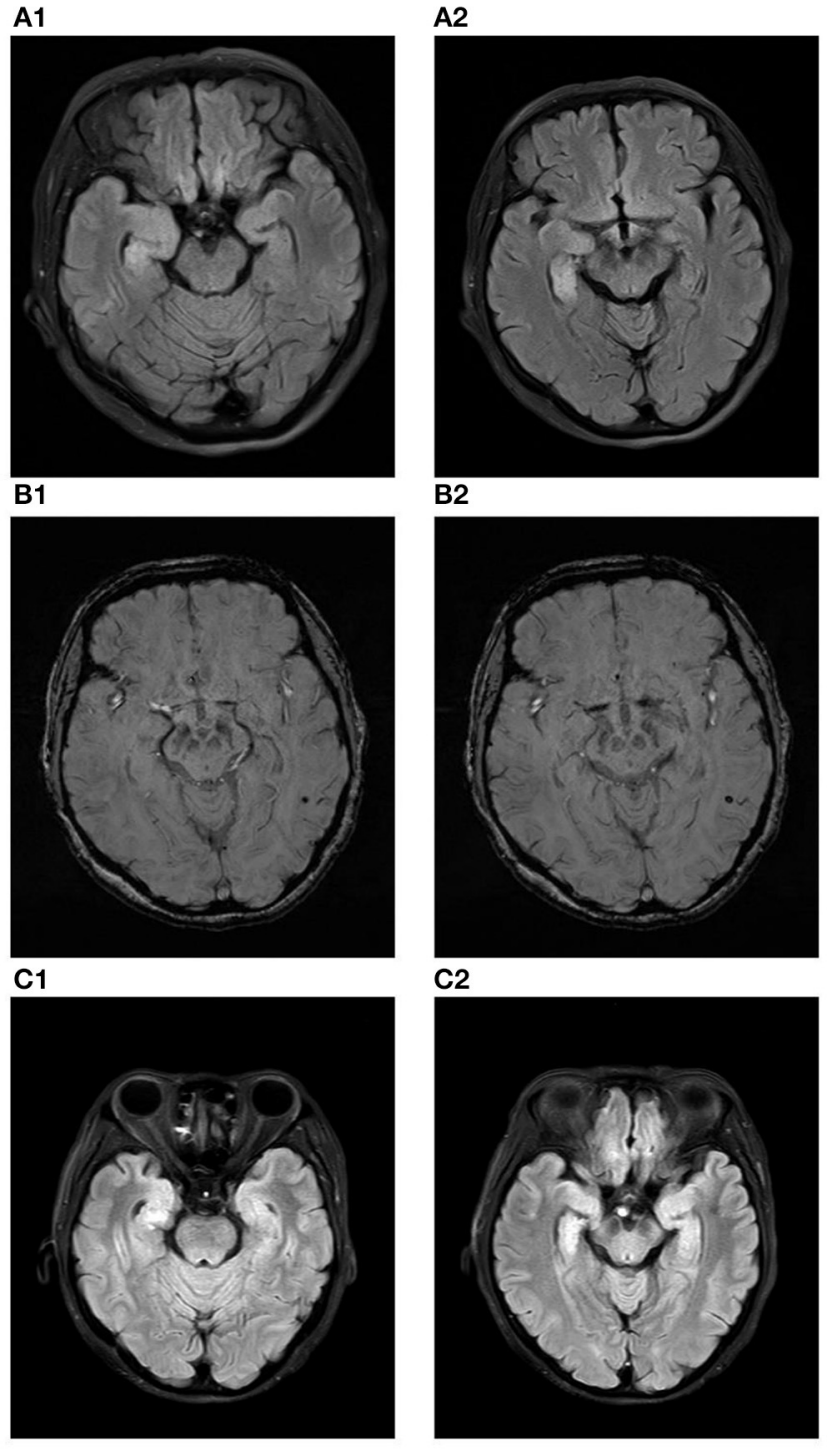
(A1,A2) show the increased volume of the right hippocampus and hyperintensities in the fluid attenuated inversion recovery (FLAIR) sequence at the time of initial diagnosis. (B1,B2) show microbleed in the left temporal lobe in the susceptibility weighted imaging (SWI) sequence at the time of initial diagnosis. (C1,C2) show the increased volume of the right hippocampus and hyperintensities in the fluid attenuated inversion recovery (FLAIR) sequence ten months after diagnose.
